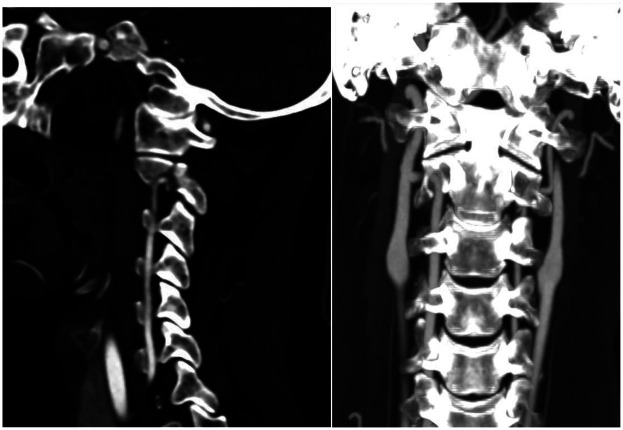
Preoperative cervical angiography CT sagittal and coronal reconstruction. Vertebral arteriography showed that the distance from the top of vertebral artery foramen to the upper articular surface was less than 2 mm, and the left side was the dominant artery.
